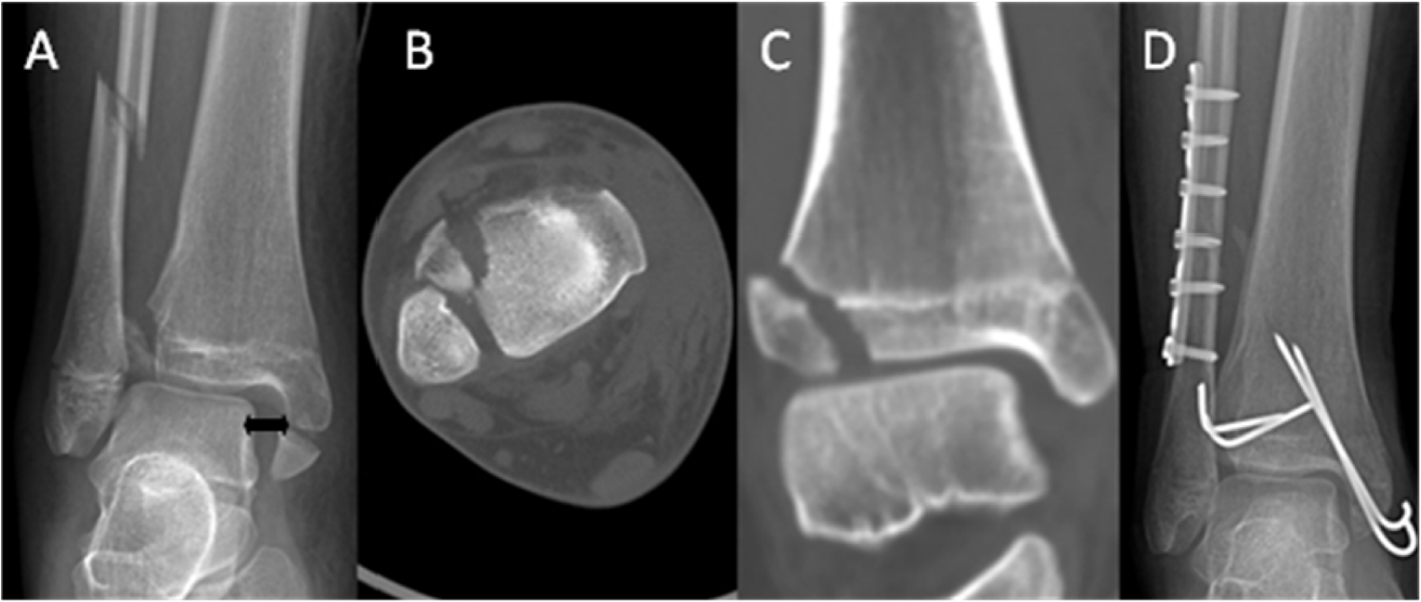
(Case 2): a AP radiograph showed Tillaux fracture, distal fibular fracture, and MMF associated with widening of the tibiofibular clear space and medial space of the ankle (black double arrow). b Axial CT section showed 8 mm displacement of Tillaux fragment and a normal incisura fibularis. c Coronal CT scan showed widening of the medial clear space of the ankle, which indicates TS. d AP plain radiograph obtained 12 months after treatment showed complete reduction of medial joint space immediately post operatively
